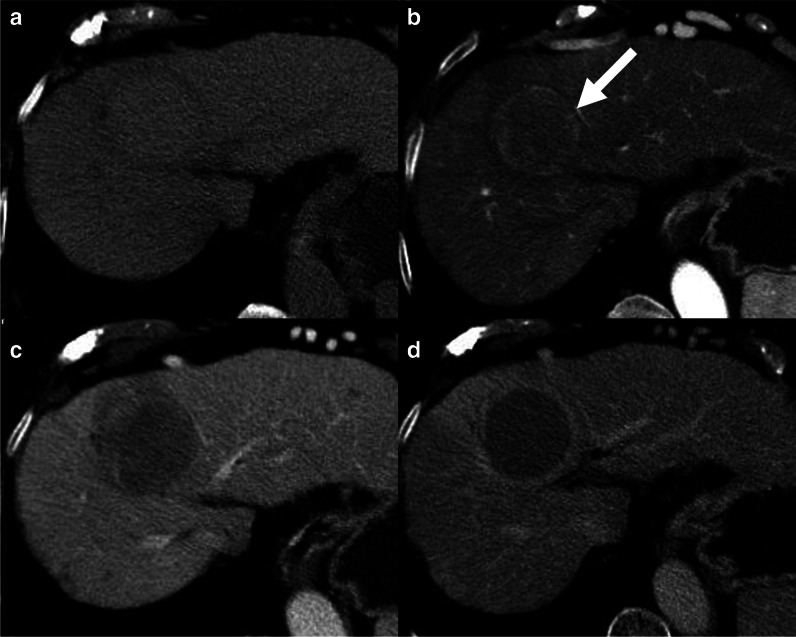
A 75-year-old woman with cirrhosis and hepatocellular carcinoma. Contrast-enhanced CT shows a lesion isodense on pre-contrast (a), with non-rim arterial phase hyperenhancement (b, arrow), non-peripheral washout on portal venous (c) and delayed (d) phases. Enhancing capsule is also visible on the delayed phase
